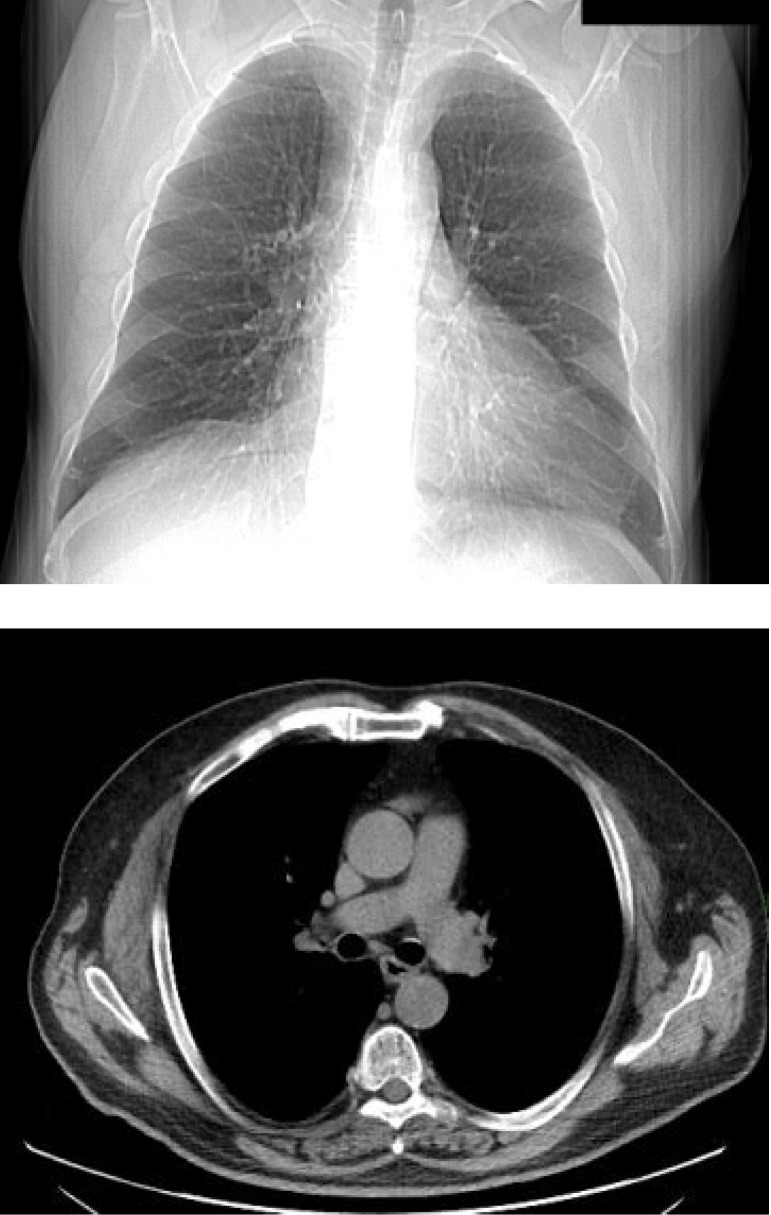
CT-scan of the thorax reveals no pathologic masses (Personal photographic archive)
